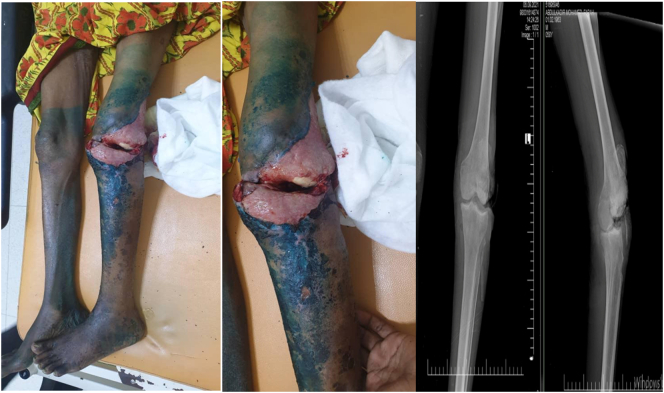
Preoperative clinical pictures and radiographs of the knee demonstrates reduced but severely infected anterior wound of the knee.
